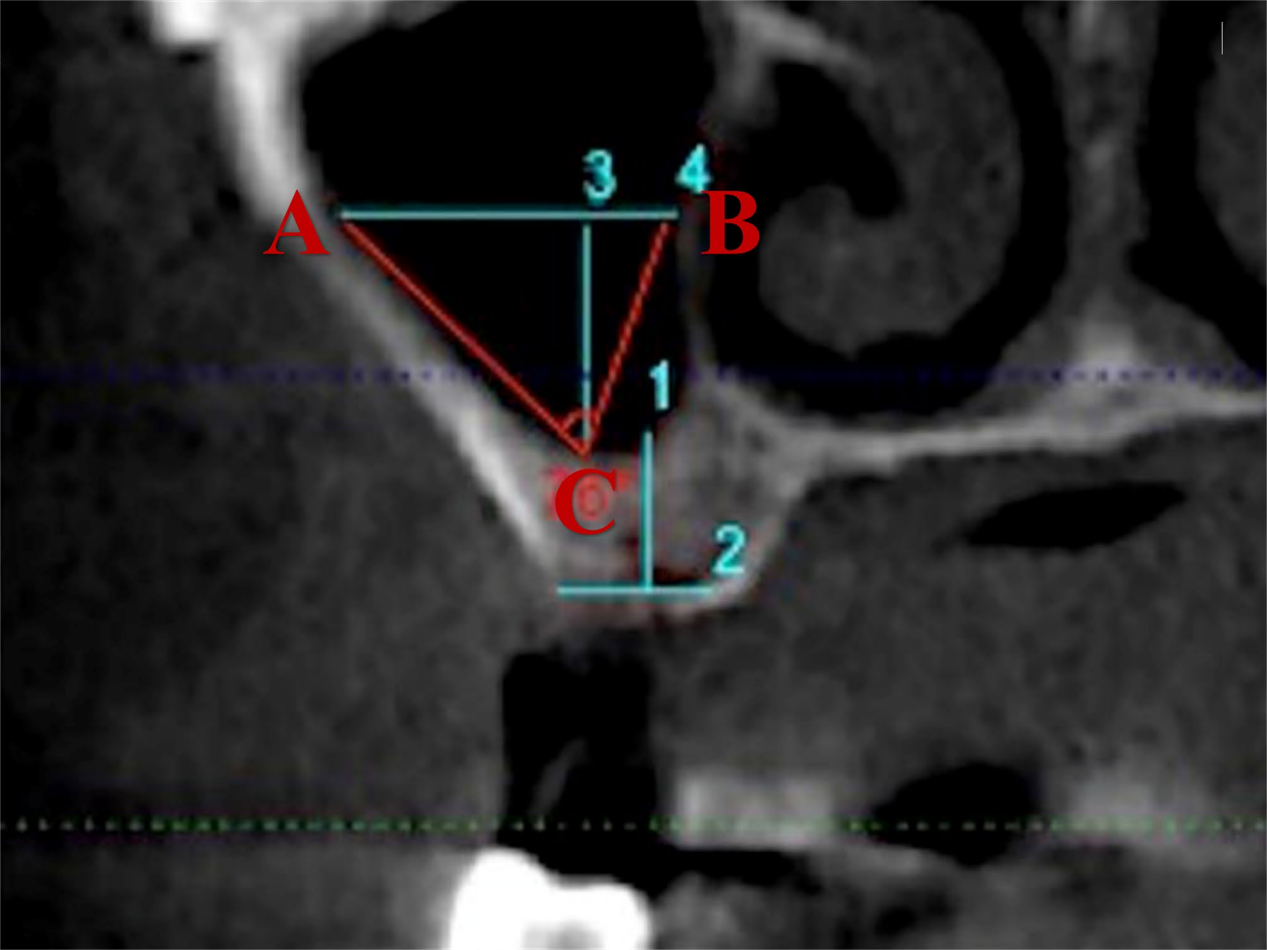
The investigated anatomical parameters [27] measured on coronal preoperative CBCT. Maxillary sinus width (L_AB_): Horizontal distance between the buccal and palatal walls of the maxillary sinus at 10 mm above the lowest point of the maxillary sinus floor at the intended implantation site. Maxillary sinus angle (∠ ACB): Angle between a horizontal line drawn 10 mm above the lowest point of the maxillary sinus floor at the intended implantation site and the buccal wall bone plate and palatal wall bone plate of the maxillary sinus
